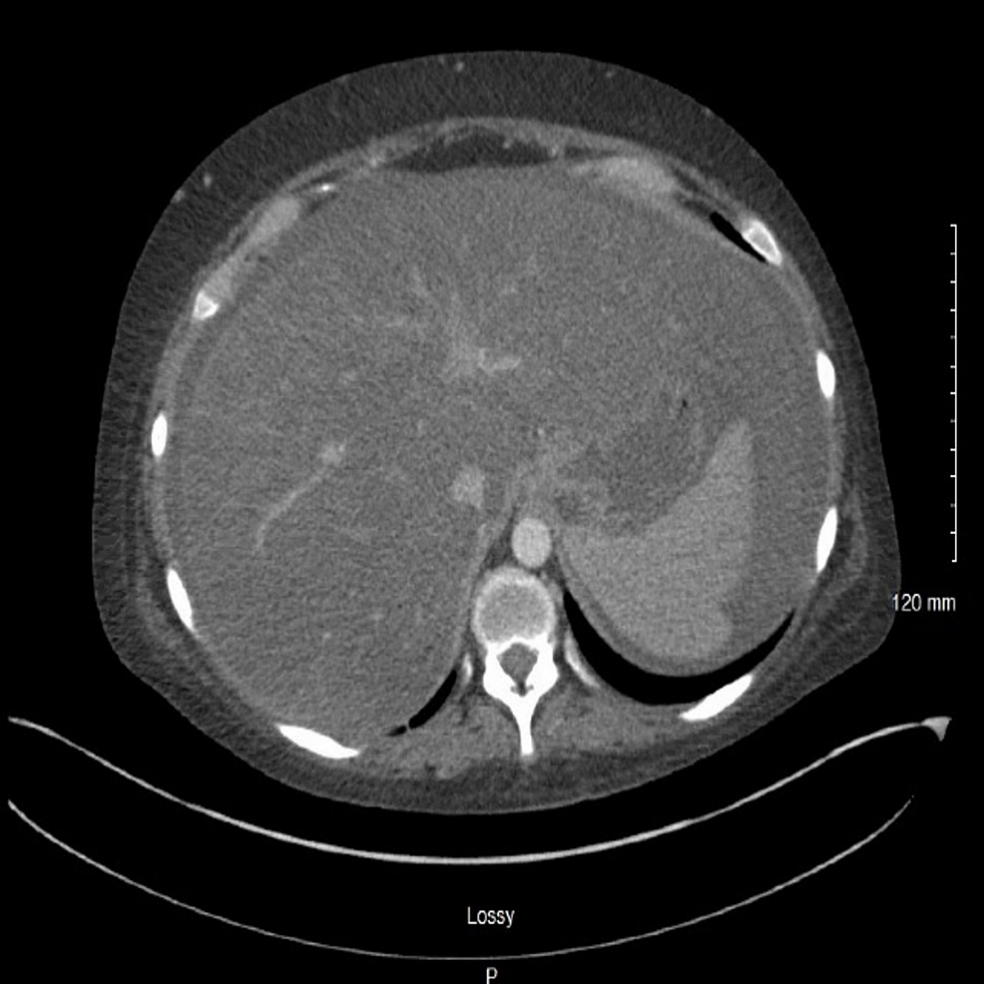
Abdominal CT demonstrates an enlarged liver, splenomegaly with a moderate volume of ascites, and cholelithiasis CT: computed tomography
